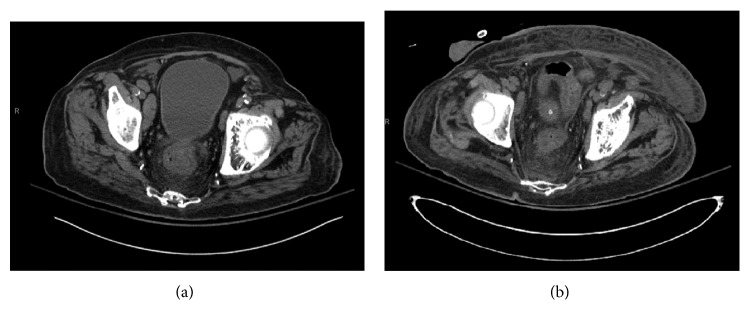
Top normal-sized pelvic lymph nodes decreased in size in repeat CT (4(b)) compared to previous CT (4(a)). The largest right external iliac lymph node measures 1.8 × 0.7 cm.
